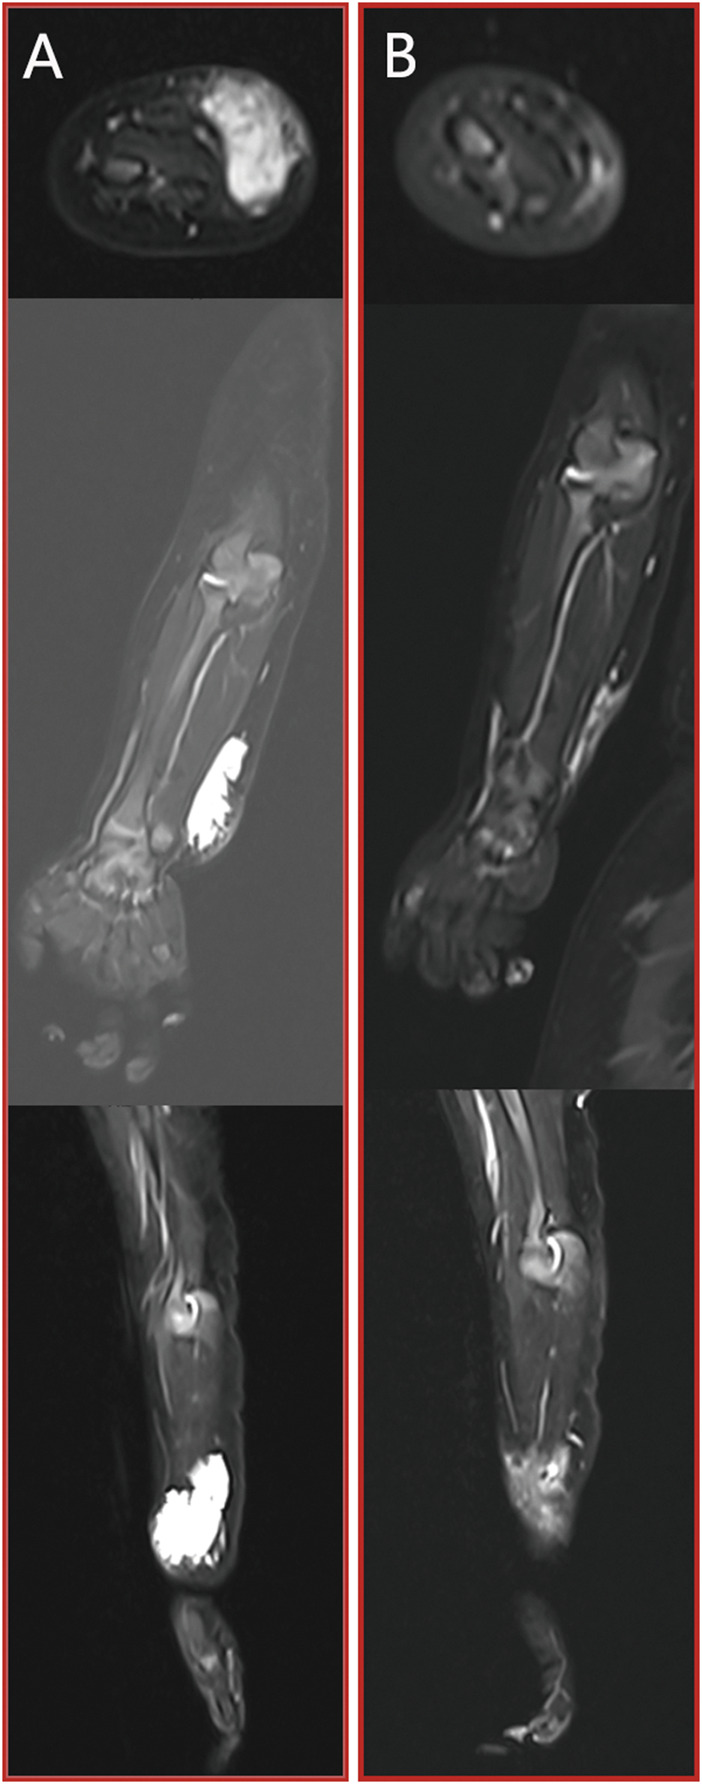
Case 3: patient with microcystic LMs of the right upper medial wrist joint which achieved a marked reduction in the size of the lesion after two sessions of combination treatment and surgical resection. (A) pre-treatment MRI stud; (B) post-treatment MRI study. From top to bottom: axial T2 fat saturated image, coronal T2 fat saturated images and sagittal T2 fat saturated images.
